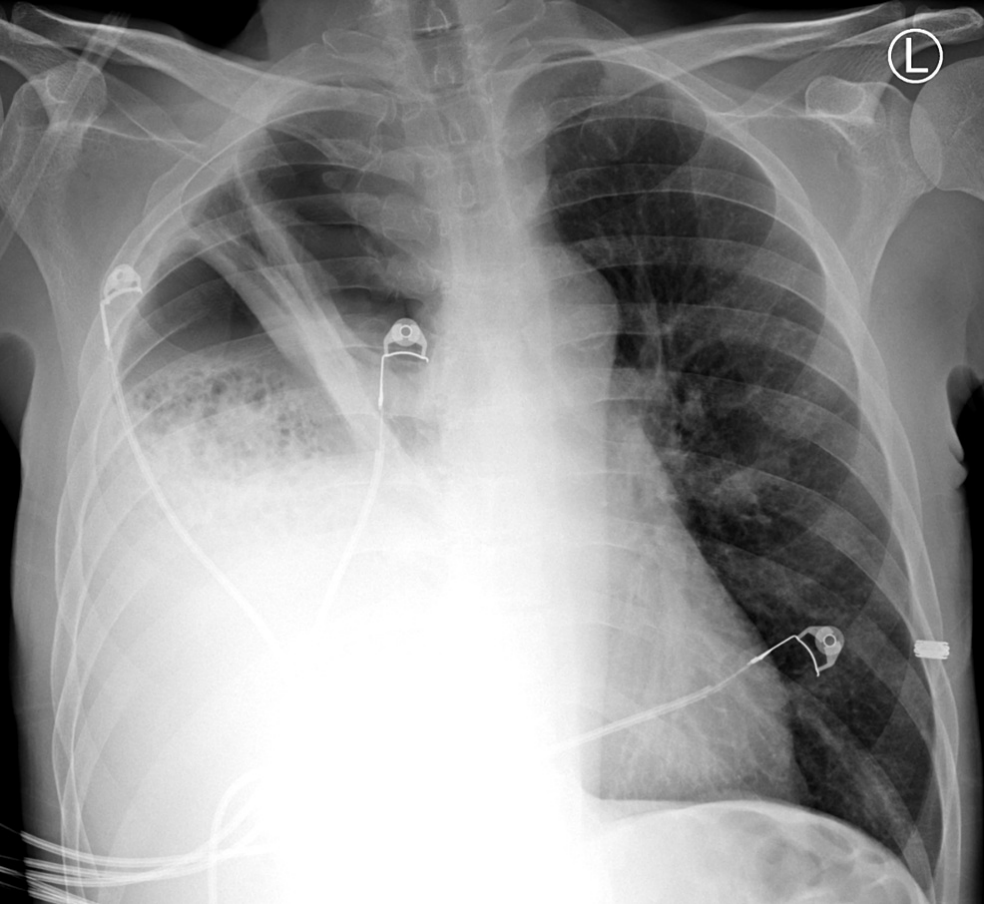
A posteroanterior (PA) chest radiograph showing bubbly lucencies in the right midlung and extensive consolidation
